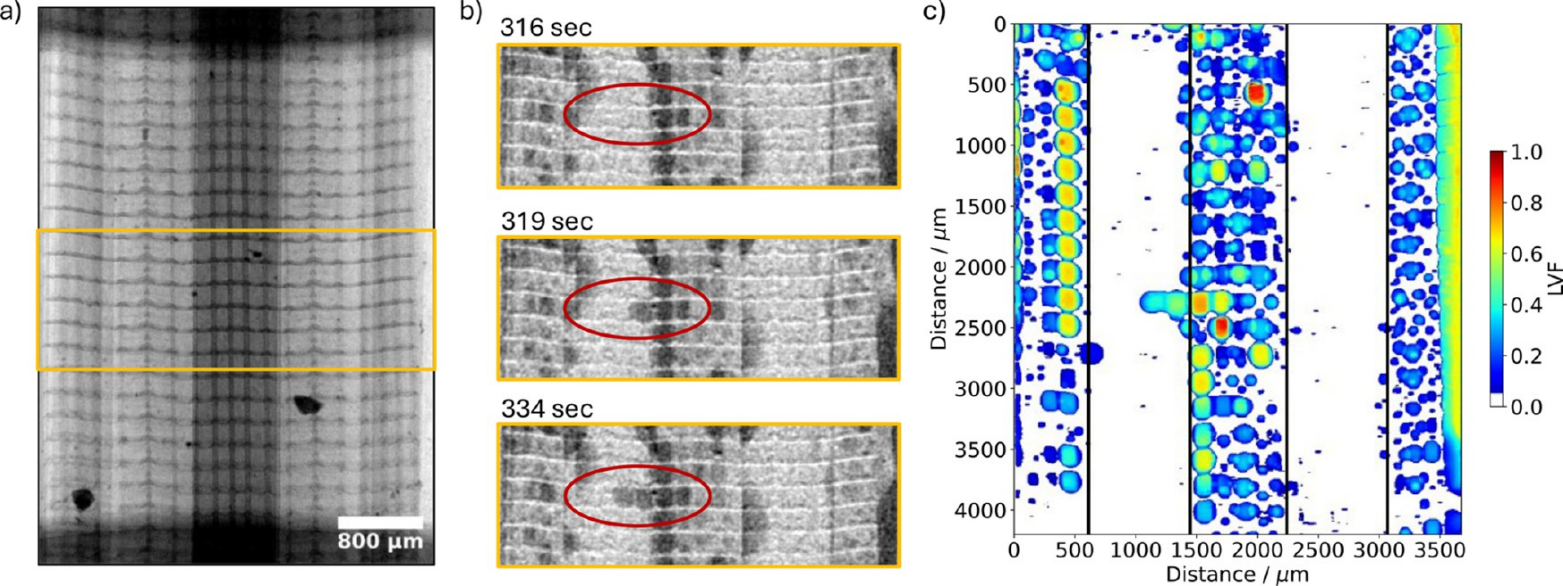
a) Radiographic projection (perspective
